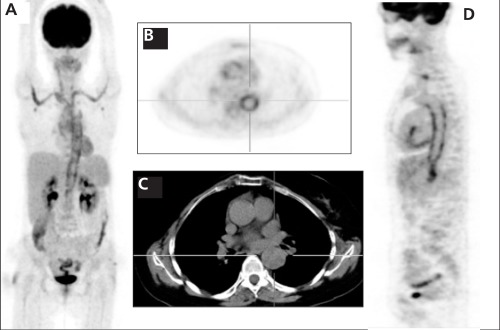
A 64-year-old woman with active Takayasu arteritis. Figure1A (MIP), Figure 1B (Axial PET), Figure 1D (Sagittal PET) imagesdemonstrated intense F 18 FDG uptake in the aorta, bilateral subclavianand brachiocephalic arteries consistent with Takayasu arteritis. The maximum standardized uptake value of the aorta was 9.2 beforetherapy
